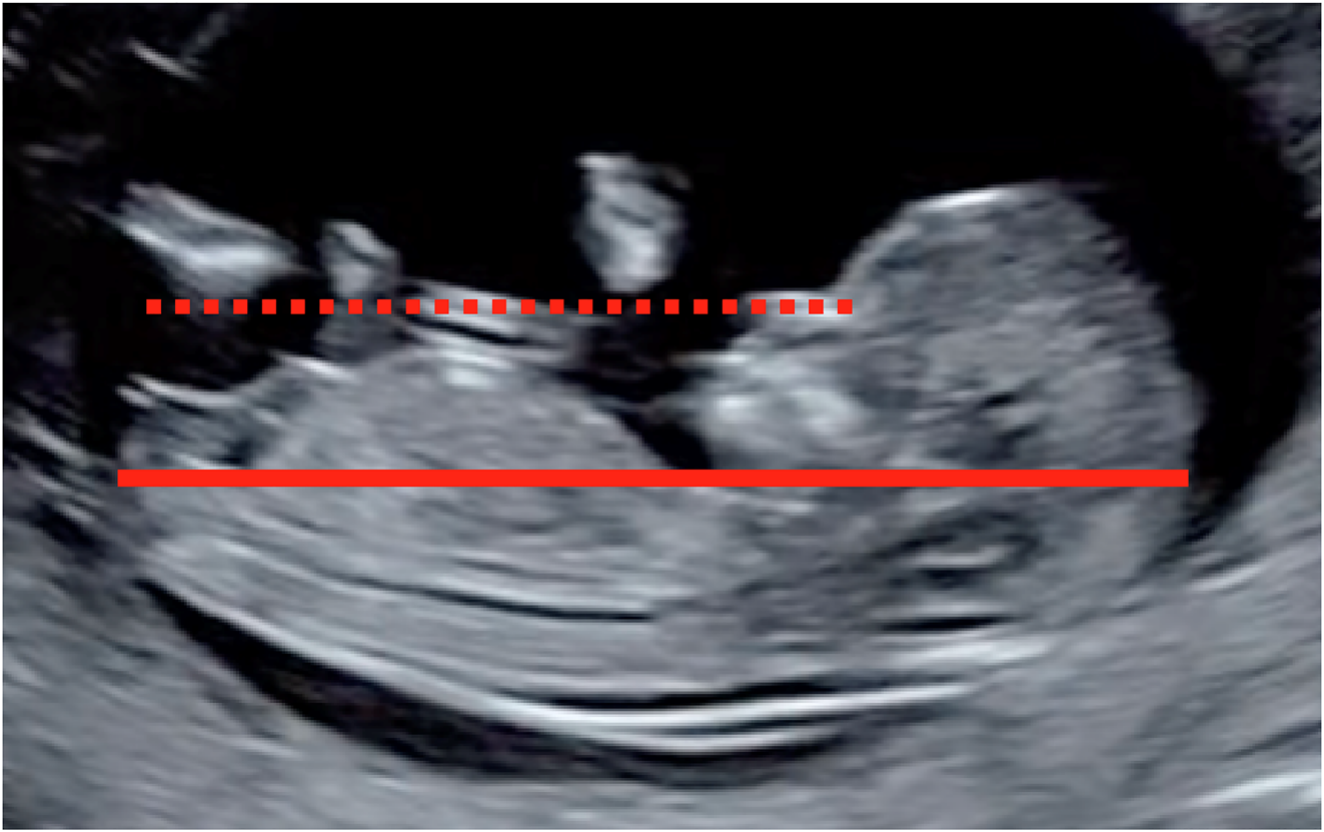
Criterios estándar para la medición de la LCR. La valoración puede realizarse por vía abdominal o vaginal. LCR entre 45 y 84 mm. Plano mediosagital: Sección sagital del feto con la cabeza alineada con el cuerpo. Debe visualizarse la punta ecogénica de la nariz, el hueso nasal si está presente, la forma rectangular del paladar, el diencéfalo, (no debe incluir la órbita), la inserción del cordón umbilical, la vejiga y el tubérculo genital. Los miembros inferiores no deben ser visibles. Correcta visualización del polo cefálico y caudal, con identificación de la coronilla, la rabadilla y la piel que los recubre. Posición fetal neutral, ni flexionada (debe identificarse una bolsa de líquido amniótico al menos equivalente a la anchura del paladar entre la barbilla y el tórax); ni extendida (ángulo del paladar entre 30–60° respecto a la horizontal). Orientación: el plano de la LCR debe estar entre 0–30° respecto a la horizontal de tal manera que la línea de medición de la LCR forme un ángulo de 90° con el haz de ultrasonidos. Una forma de asegurar la posición horizontal del feto es trazar una línea desde la punta de la nariz, que ha de estar al nivel o por encima de la pared abdominal en relación con la horizontal. Magnificación: la sección completa de la LCR debe ocupar más del 60% de la pantalla y debe incluirse en su totalidad. Colocación correcta de los cálipers cubriendo la máxima distancia entre el borde externo de coronilla y la rabadilla. Debe medirse al menos en 3 ocasiones y registrar la medida mayor que cumpla los criterios adecuados.
